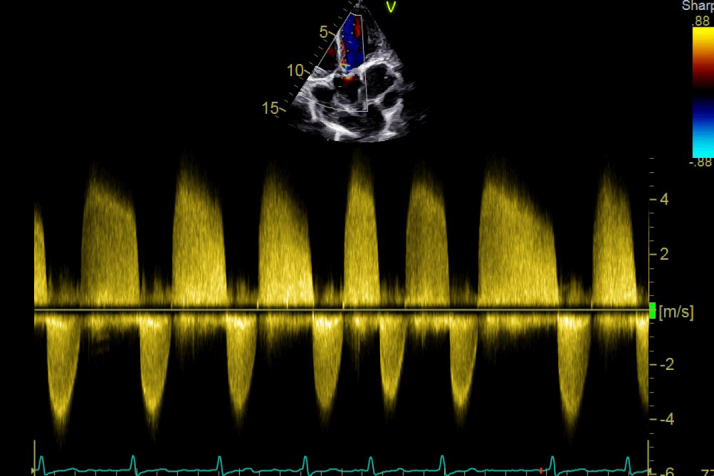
2D-TTE Continuous-wave Doppler tracing across the aortic valve showing a peak velocity of 4.1 m/s. s/o = severe aortic stenosis.
